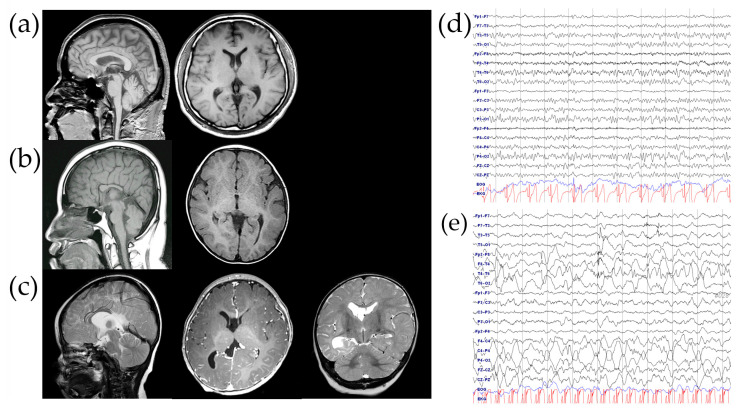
The results of brain magnetic resonance imaging (MRI) and an electroencephalogram (EEG). (a–c) A brain MRI exhibited normal findings in the proband’s grandmother (a); a hypoplastic corpus callosum with absent rostrum, splenium, and posterior body in the proband’s mother (b); and dysgenesis of the corpus callosum with migration abnormality in the proband. (d,e) The EEG showed normal findings in the proband’s mother (d) but abnormal EEG findings, with sharp and slow waves in the right temporo-occipital areas in the proband (e).
